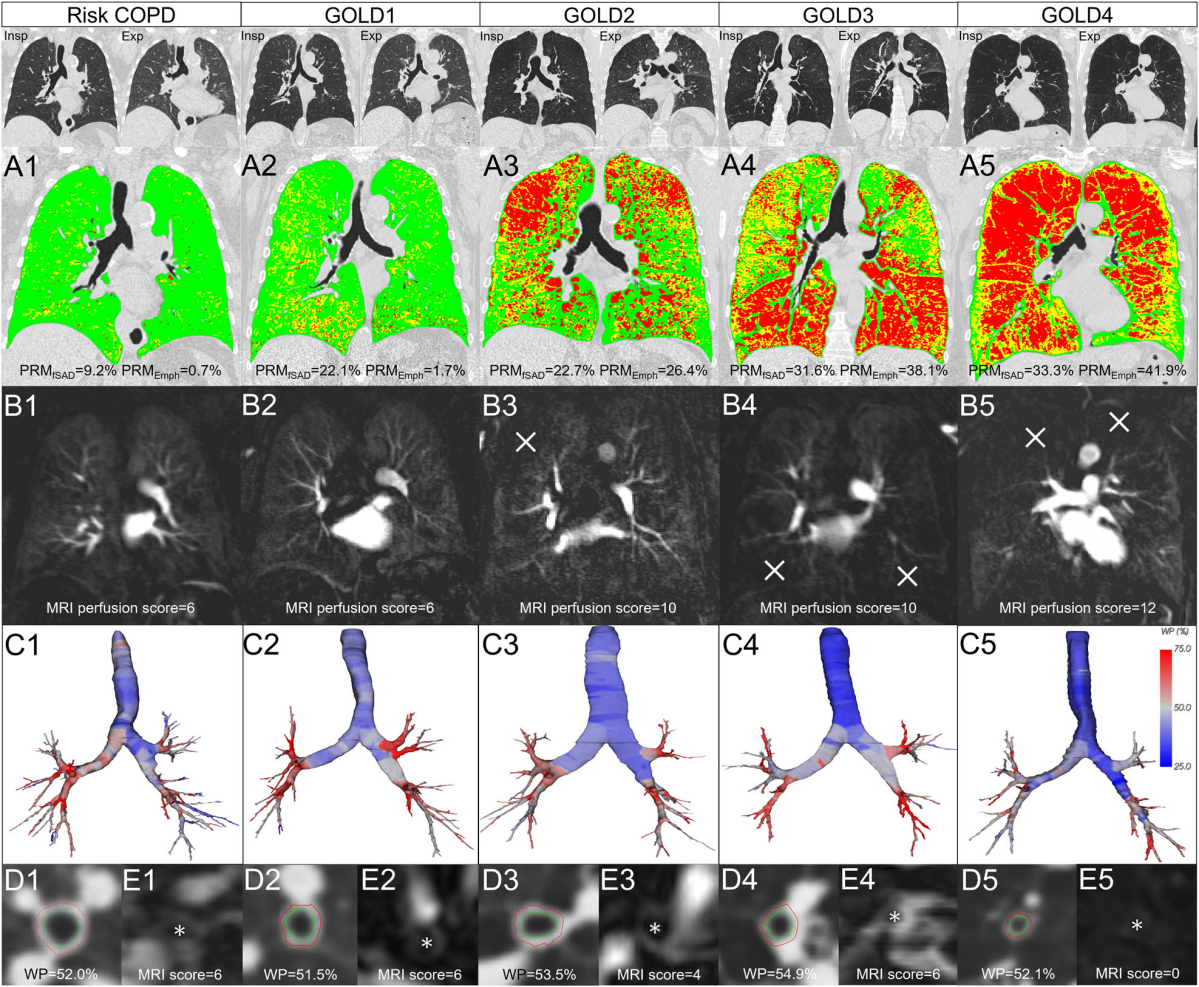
GOLD stage-specific imaging characteristics in QCT and MRI. A1–A5 Quantitative CT images with parametric response imaging (PRM) with normal lung (green = PRM_Normal_), functional SAD (yellow = PRM_fSAD_), and emphysema (red = PRM_Emph_). Note: air trapping and emphysema increase with the GOLD stage. B1–B5 Perfusion MRI images showing homogeneous lung signal in GOLD1 and extensive perfusion defects (white cross) in GOLD4. Note: MRI perfusion defects correspond to CT emphysema. C1–C5 Segmented airway tree on CT (blue = low WP and red = high WP). D1–D5 Axial images of lobe bronchi on CT with segmented airway walls with the highest WP in GOLD3. E1–E5 Transverse T1 VIBE images of lobe bronchi on MRI. Note: assessment of the airway with MRI is much more challenging than with CT
